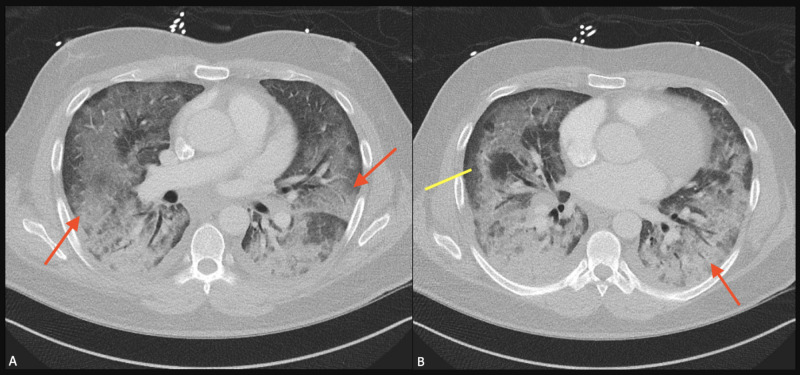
Computed tomography of the chest on Day 3 of admission showed (A) rapidly worsening bilateral lower lobe infiltrates (red arrows) with (B) subpleural sparing (yellow line) and worsening lower lobe infiltrate (red arrow).
